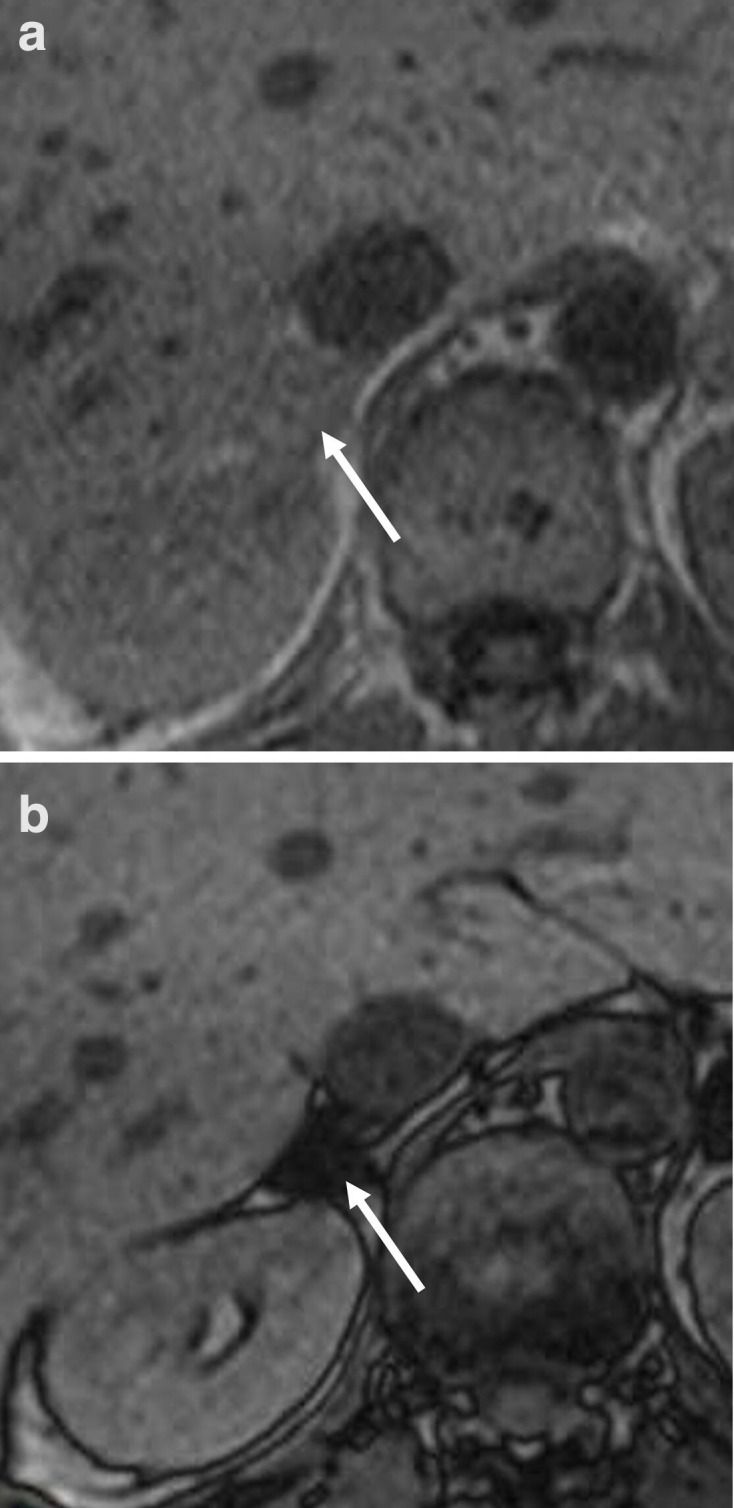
Axial a) in and b) opposed phase MRI images showing a right sided adrenal adenoma (signal drop in the opposed phase comared to the in phase.
